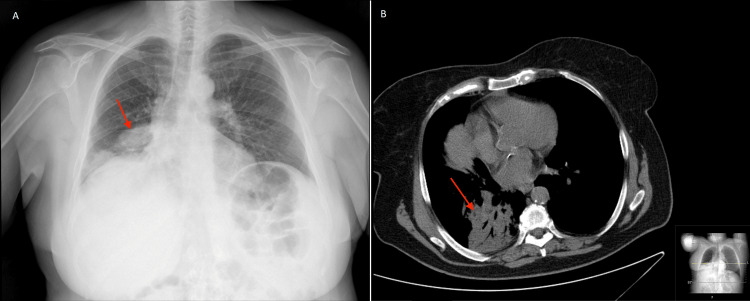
Chest X-ray (A) and CT scan (B) on admission showing lower right lobe consolidation with air bronchogram, suggestive of acute infection (red arrow).
